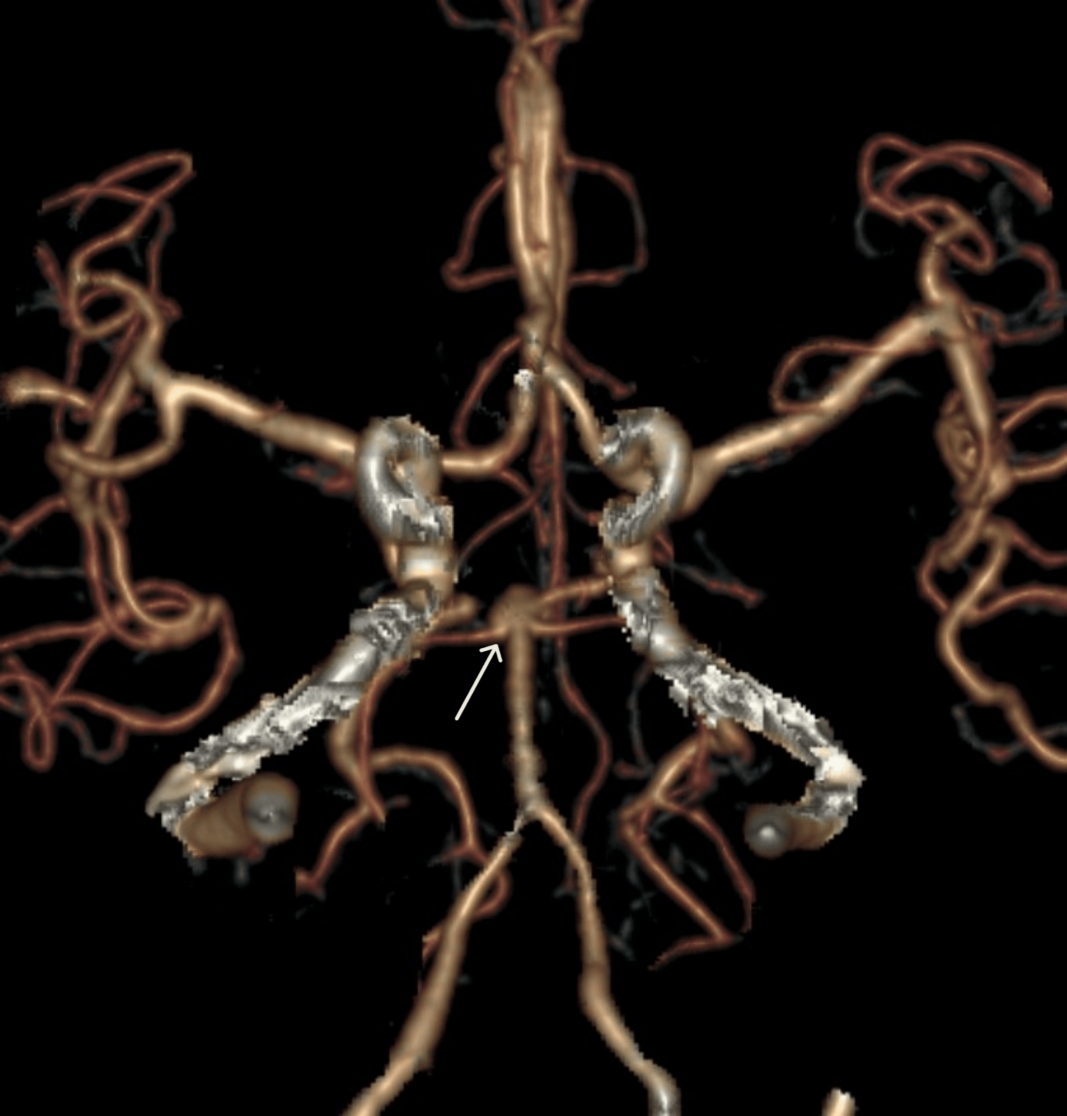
Coronal CT angiography VR image demonstrating a fusiform aneurysm at the top of the basilar artery (white arrow) CT: computed tomography, VR: volume rendering
